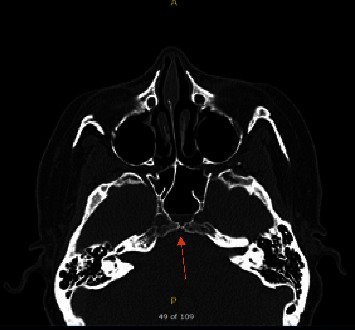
CT sinus without contrast axial cut showing the air-fluid level in the sphenoid sinus and defect in the clivus (see arrow).
